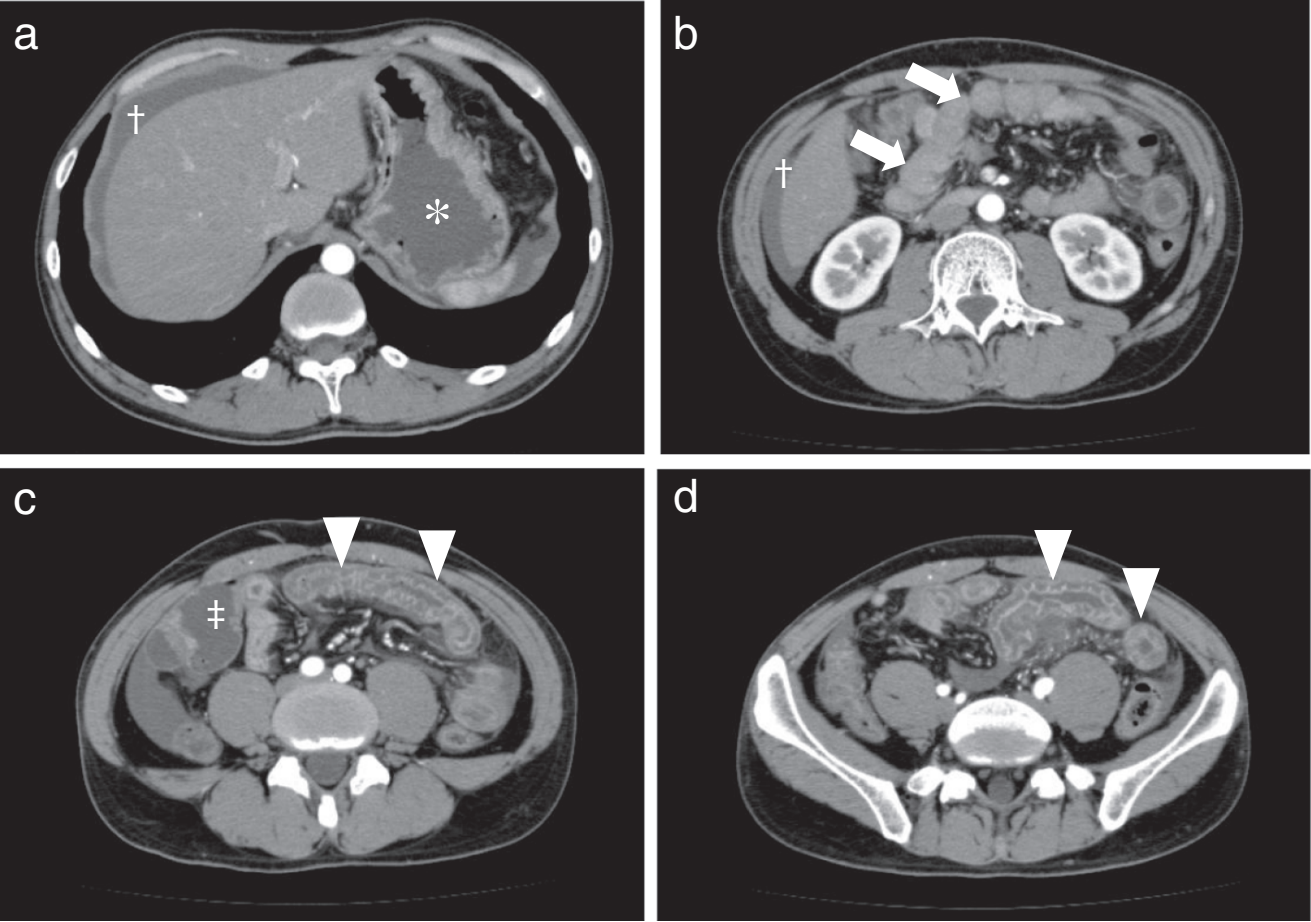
Enhanced abdominal computed tomography (A-CT) of severe gastrointestinal edema. a: Liver-stomach level slice, b: Kidney level slice, c: Under umbilical level slice, d: Pelvis level slice. Patient No. 3 visited the emergency department because of severe abdominal pain and vomiting. Obstructive duodenum (arrow) of the small intestine (arrow head) was visualized due to the thickening of the gastrointestinal walls. Gastric (*) and intestinal walls (‡) were expanded. Moderate ascites was observed around liver (†). (Photos were provided by Dr. N. Hosoi, Tokyo-Kita social insurance hospital).
